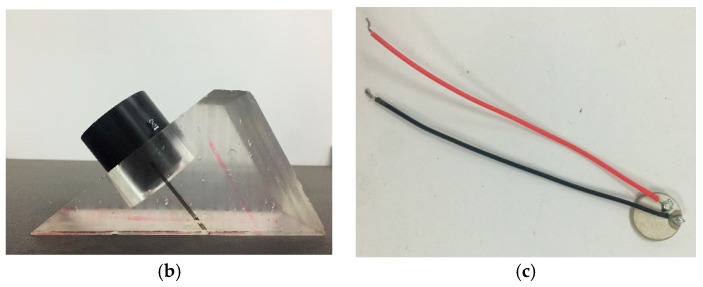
(a) The RAM-5000 SNAP nonlinear ultrasonic system; (b) Transmitting transducer; (c) Piezoelectric ceramics (the type: PSN-33).
